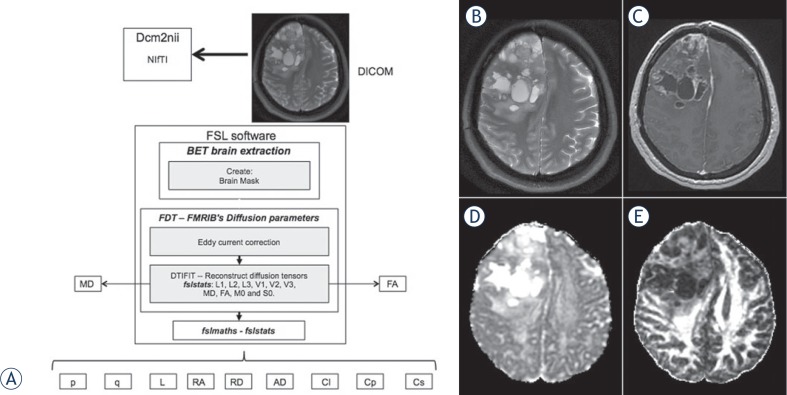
(A), FSL software algorithm used in the image postprocessing and data analyses. (B–E), Examples of acquired sequences in a patient with GBM and the tensor-metric maps generated for the data analyses: (B), axial T2-weighted; (C), post contrast axial T1-weighted; (D), axial diffusivity (AD) tensor map; and  (E),  fractional anisotropy (FA) tensor map. Notice how it might not be possible to perform an imaging diagnosis based only on a visual inspection of these maps.
